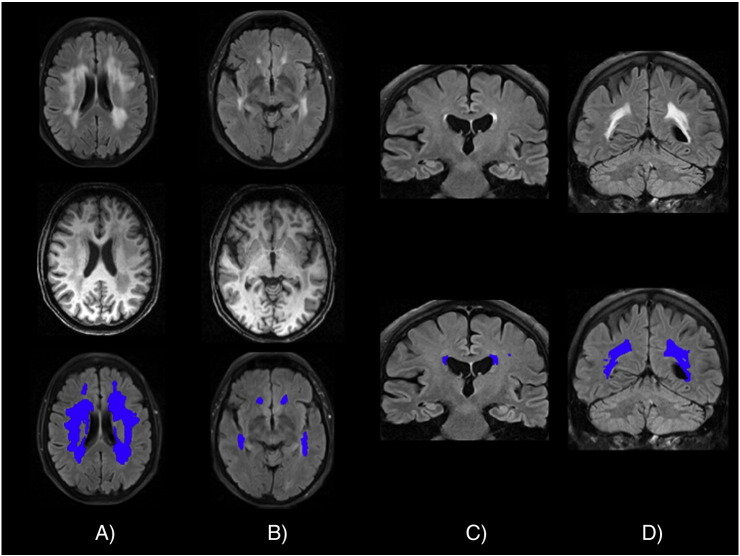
Examples of manual masks for different datasets (a,b Dataset 1; c,d Dataset 2) and WMH load (a,c low WMH load; b,d high WMH). Top row shows the FLAIR images, the bottom row shows the manual mask overlaid in blue. For Dataset 1, the corresponding T1w image is shown in the middle row.
